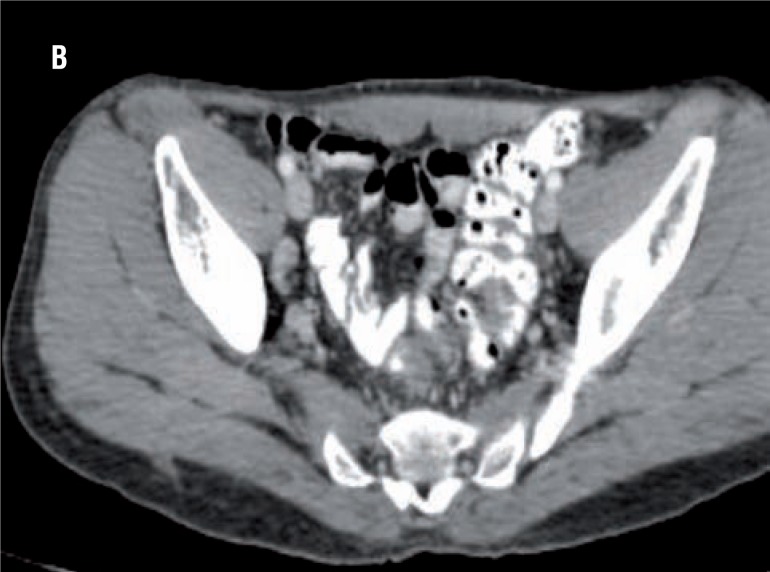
CT showing enlarged lymph node in the pelvis.
